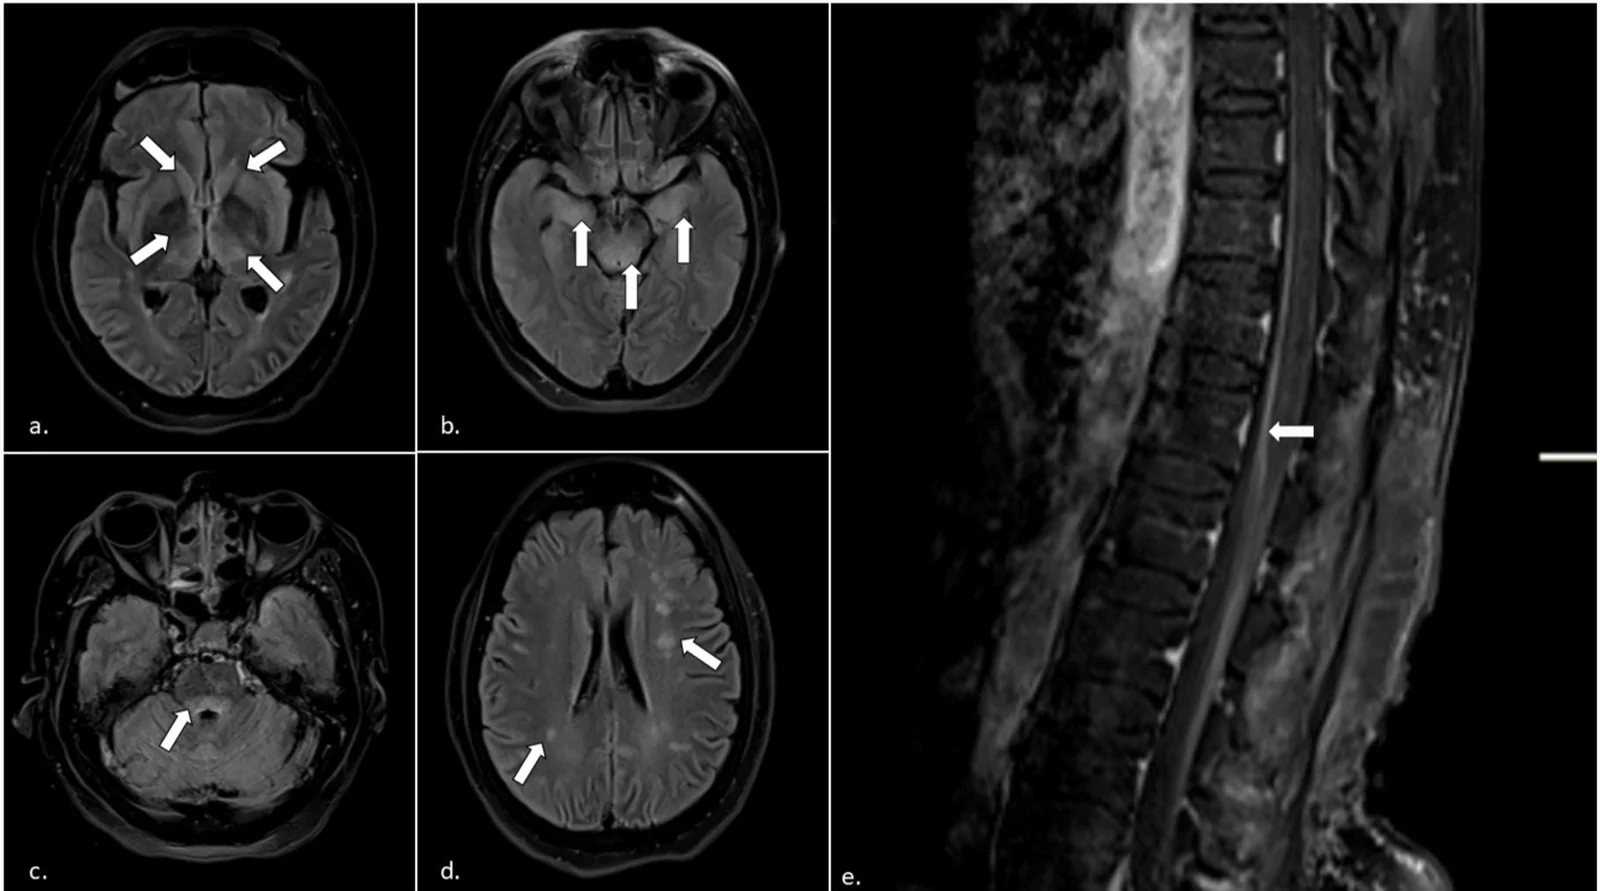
Magnetic resonance imaging of the brain and spine. (a) Axial fluid-attenuated inversion recovery (FLAIR) image at the level of the basal ganglia showing hyperintensity involving the heads of the bilateral caudate nuclei, thalami and putamina (arrows). (b) Axial FLAIR image at the level of the midbrain showing hyperintensity involving the dorsal aspect of the midbrain and bilateral hippocampi (arrows). (c) Axial FLAIR image at the level of the pons showing hyperintensity involving the dorsal pons (arrows). (d) Axial FLAIR image showing bilateral asymmetrical white-matter FLAIR hyperintensities involving the bilateral corona radiata and periventricular white matter (arrows). (e) Post-contrast sagittal image at the level of the conus showing enhancement along the anterior aspect of the conus (arrows).
